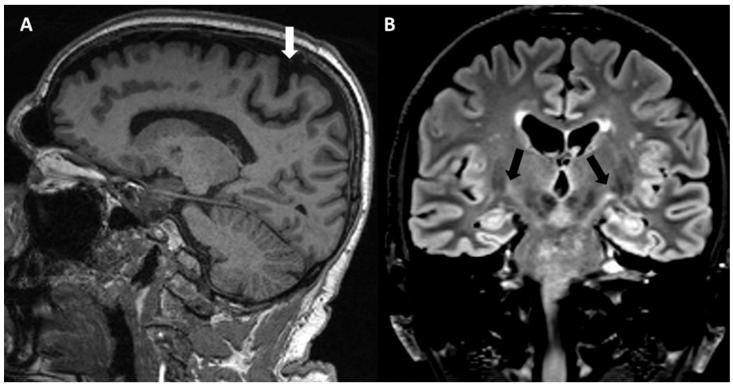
MRI in a PLS patient. (A) Sagittal T1 sequence showing atrophy of primary motor cortex with enlargement of central sulcus (white arrow). (B) Coronal flair sequence showing bilateral corticospinal tract hyperintensities (black arrows).
